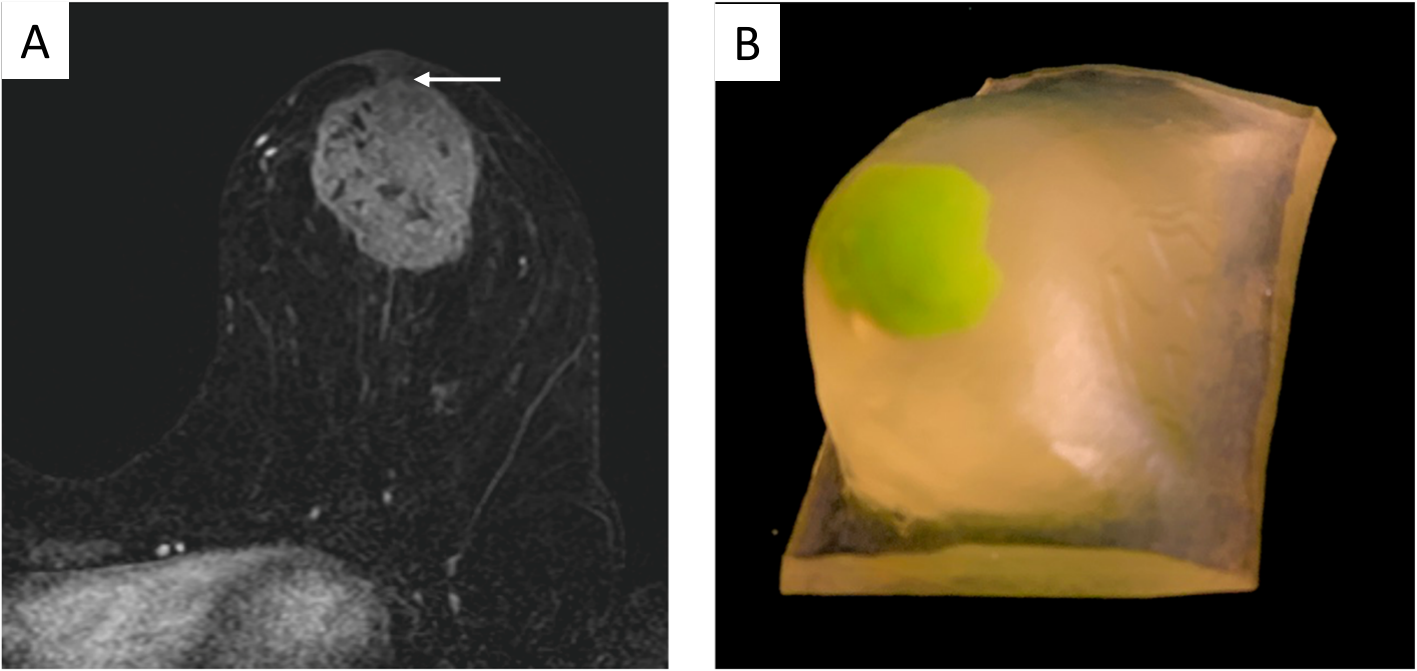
a Axial contrast-enhanced MRI showing a left breast mass with direct invasion of the toverlying nipple areolar complex (arrow). b A patient-specific 3D printed model generated from the MRI was used for patient education and obtaining of informed consent. 3D printed models allow patients to easily understand the extent of disease and rationale for a specific surgical approach
